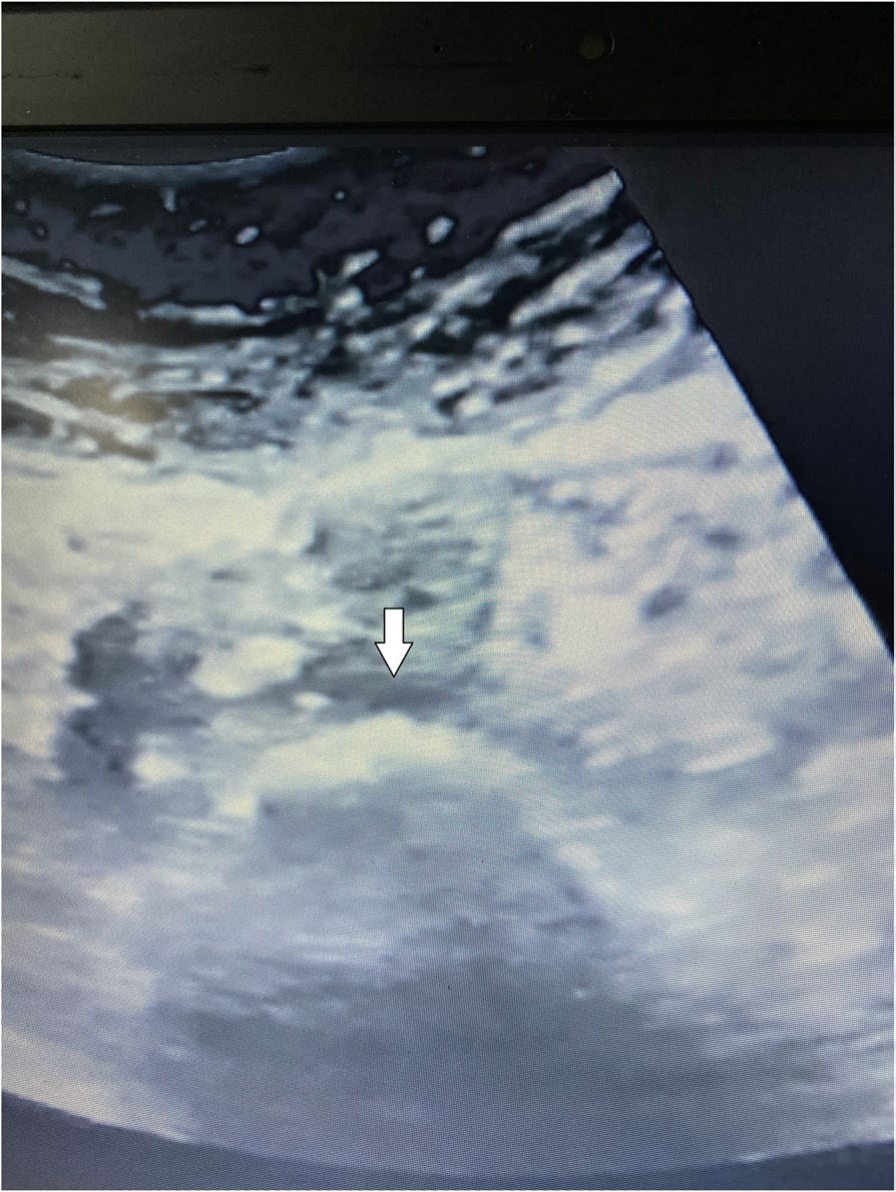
Ultrasound view showed the phenol in front of the body of the fifth lumber vertebra (white arrow)
